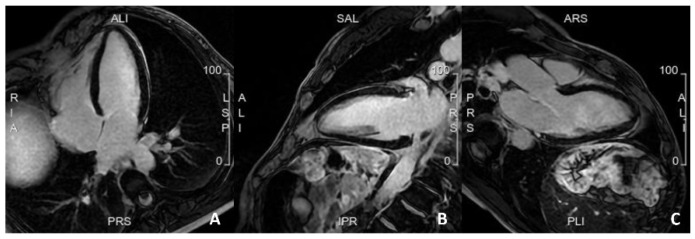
A case of a patient with a family history of sudden cardiac death, with frequent but isolated ectopic ventricular beats. Cardiac MRI was completely normal, with no evidence of myocardial fibrosis. (A). 4 Chamber view; (B). 2 Chamber view; (C). 3 Chamber view.
